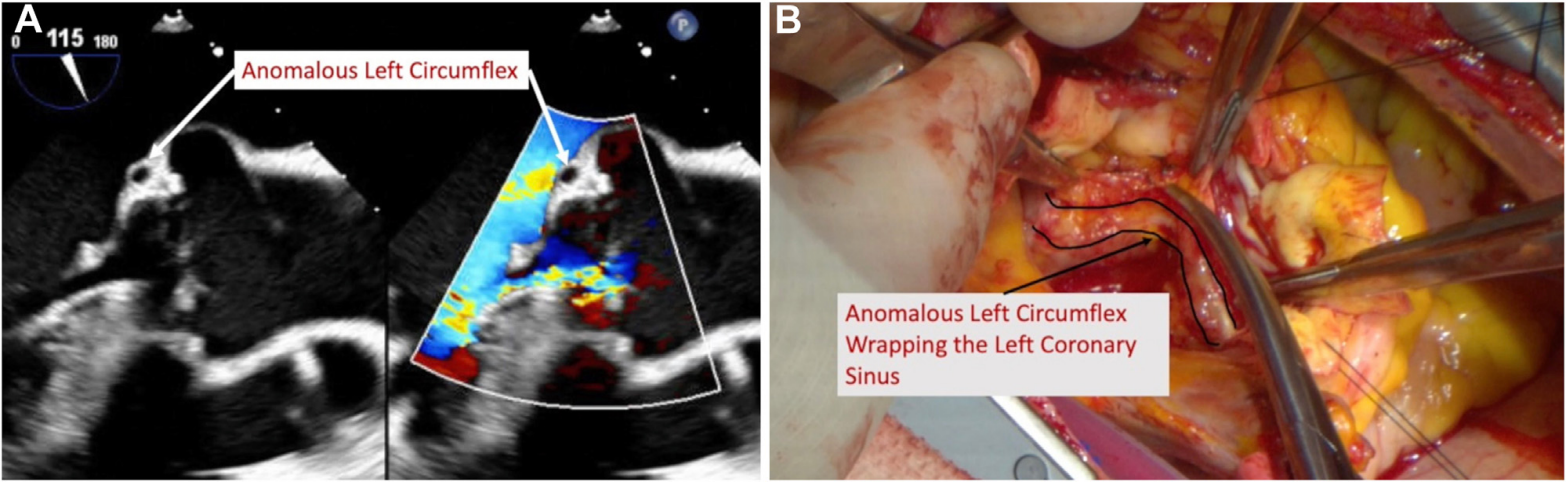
(A) Intraoperative transesophageal echocardiography demonstrates the anomalous left circumflex wrapping the left/noncoronary sinus at/below the level of the annulus. (B) The anomalous left circumflex is dissected along its retroaortic course to accommodate the primary suture line.
